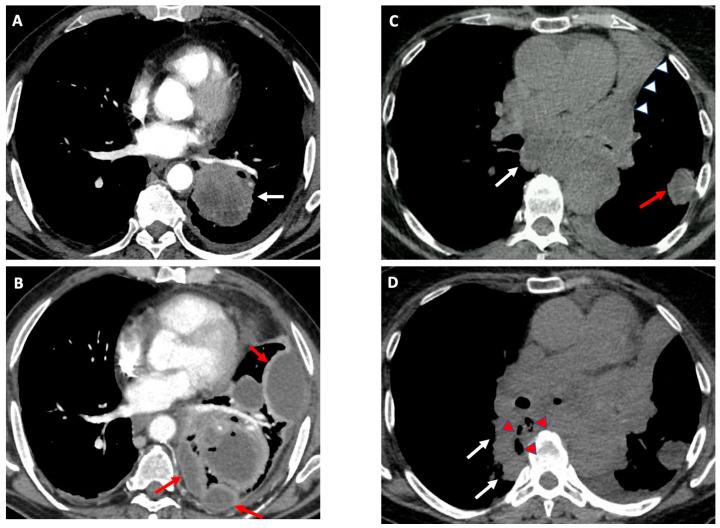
Infectious complications after endosonography: Contrast-enhanced CT scan showing a left lower lobe mass (white arrow) with low-dose attenuation (A). A marked increase in the size of the pulmonary mass and the presence of a multiloculated pleural effusion (red arrows) consistent with empyema were evident in a follow-up CT performed 12 days after an EBUS-TBNA of the pulmonary lesion (B). Unenhanced CT (patient allergic to the contrast medium) showing the complete atelectasis of the left upper lobe (with arrowheads), a subpleural nodule of the apical segment of the left lower lobe (red arrow), and an enlarged subcarinal lymph node (white arrow) (C). Fourteen days after an EUS-B-FNA of the subcarinal lymph node, a follow-up CT performed for the onset of persistent high-grade fever suggested a mediastinitis, as indicated by a marked increase in the size of the lymph node (white arrows) and the appearance of intralesional air coefficients (red arrowheads) (D).
